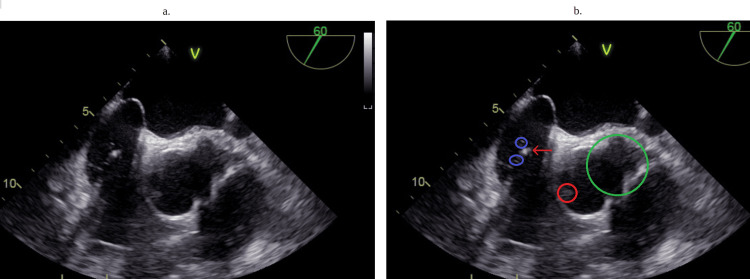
Transesophageal echocardiogram Figure 4a: shows an unmarked image of the patient's transesophageal echocardiogram. Figure 4b: shows mobile structures that are attached to leads suggestive of various vegetation along the leads of the pacemaker, which are circled in blue, while the red arrow is one of the pacemaker leads. The red circle marks the vegetation that is noted on the aortic valve, and the green circle is where an aortic aneurysm is present.
